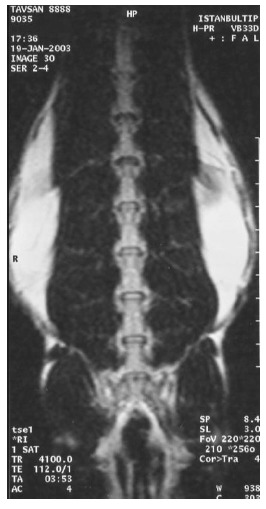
Degenerated discs on MRI.
